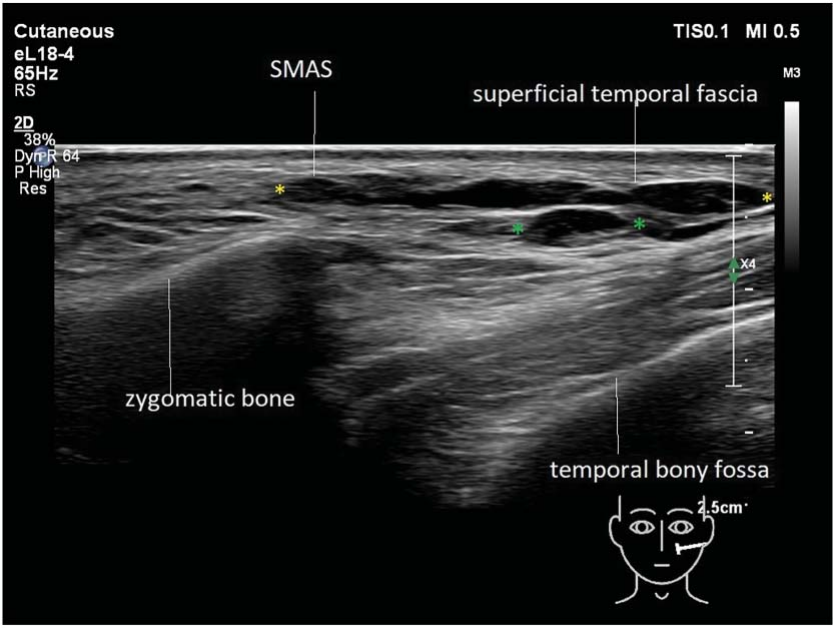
Ultrasound (grayscale, oblique view) of the temple area depicting filler material injected into the SMAS distributed into the superficial temporal fascia (yellow markers) and some filler material in the deep interfascial plane (green markers [Philips affinity 70 18 MHz]).
